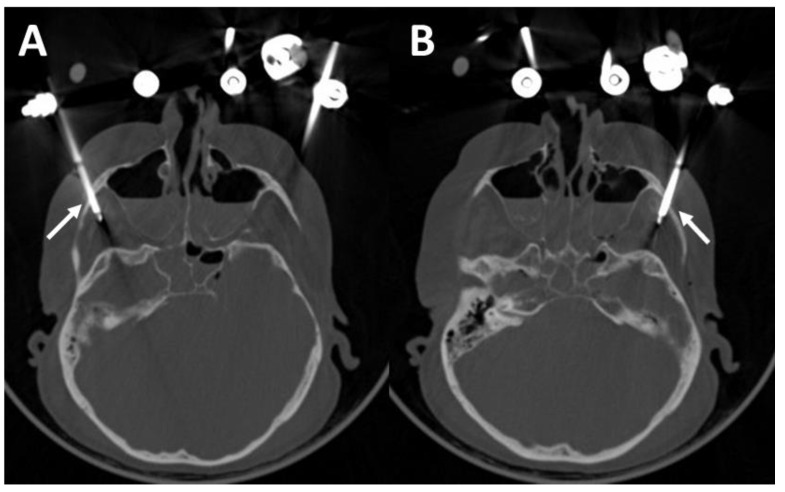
CT scans of the fractured skull with right (A) and left and right (B) zygomatic pins (white arrows).
